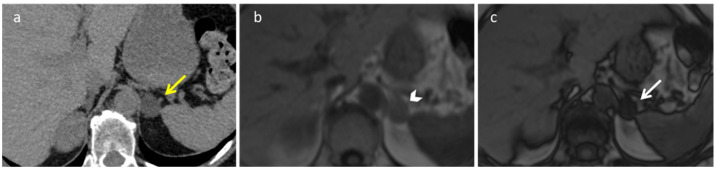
Adrenal adenoma in a 63-year-old woman. Unenhanced CT (a) shows a 2 cm left adrenal lipid rich adenoma (yellow arrow) characterized by attenuation values lower than 10 HU. Axial T1-weighted in-phase (b) and out-of-phase (c) MR images demonstrate a signal loss in the out-of-phase images (white arrow).
